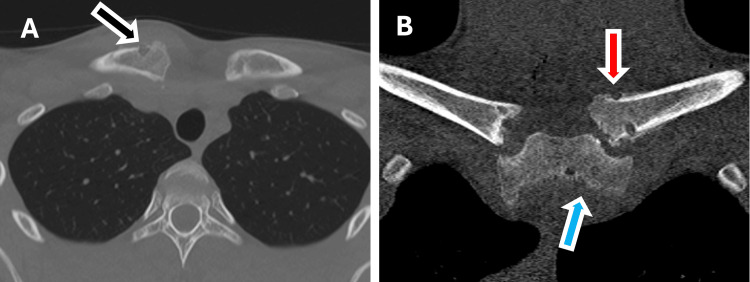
Post-operative computed tomography scan following removal of the disc and horizontal hamstring tendon autograft. A. Axial computed tomography scan demonstrating that the left clavicle is now symmetrically positioned with the right side. The superior anterior-to-posterior drill hole through the medial clavicle can be seen in the anterior cortex (black arrow). B. Coronal computed tomography scan demonstrating symmetry between the right and left clavicles. The two anterior-to-posterior drill holes can be seen in the medial clavicle (red arrow) and sternum (blue arrow).
